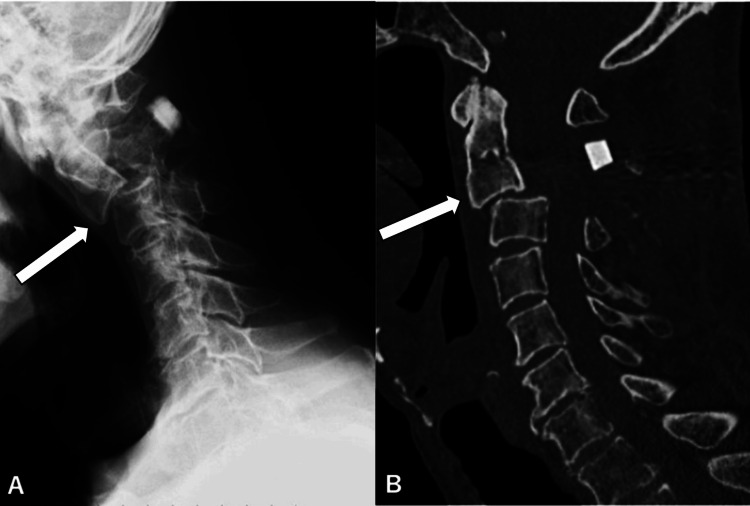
Plain X-ray (A) and CT (B) of the cervical spine on the same patient taken one week postoperatively, demonstrating subluxation at the C2/3 level, the kyphotic angle of 33 degrees (arrows).
